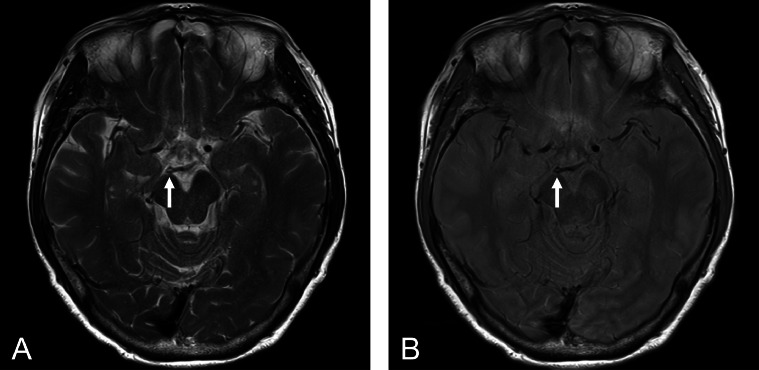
Magnetic resonance imaging (A, T2-weighted image; B, proton density-weighted image) showing fenestration around the P1-P2 segment of the right posterior cerebral artery (arrows).
